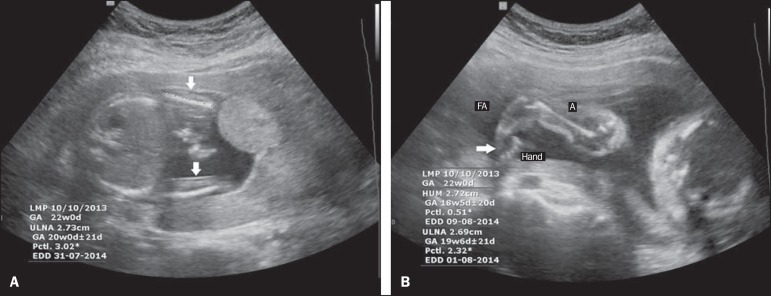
Ultrasound images of a fetus with TAR syndrome, at 21 weeks of gestation.
